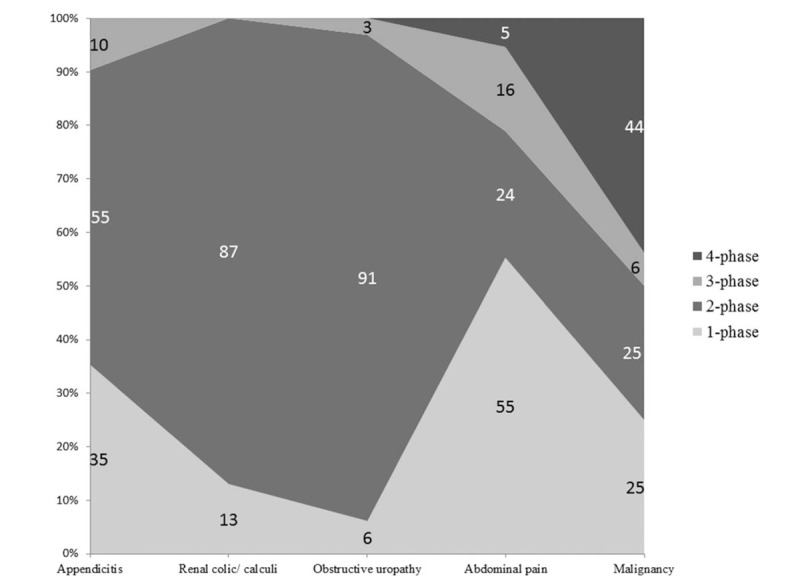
Top 5 most common clinical indications for abdomen-pelvis CT and the number of scan phases performed per patient.
